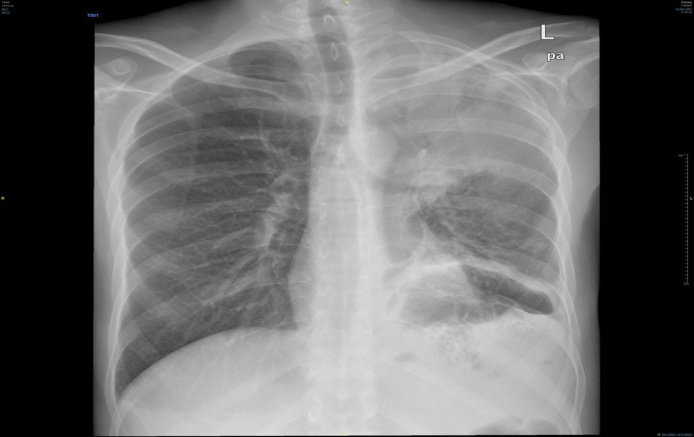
Chest x-ray reveled cavity at left side with pleural effusion.
